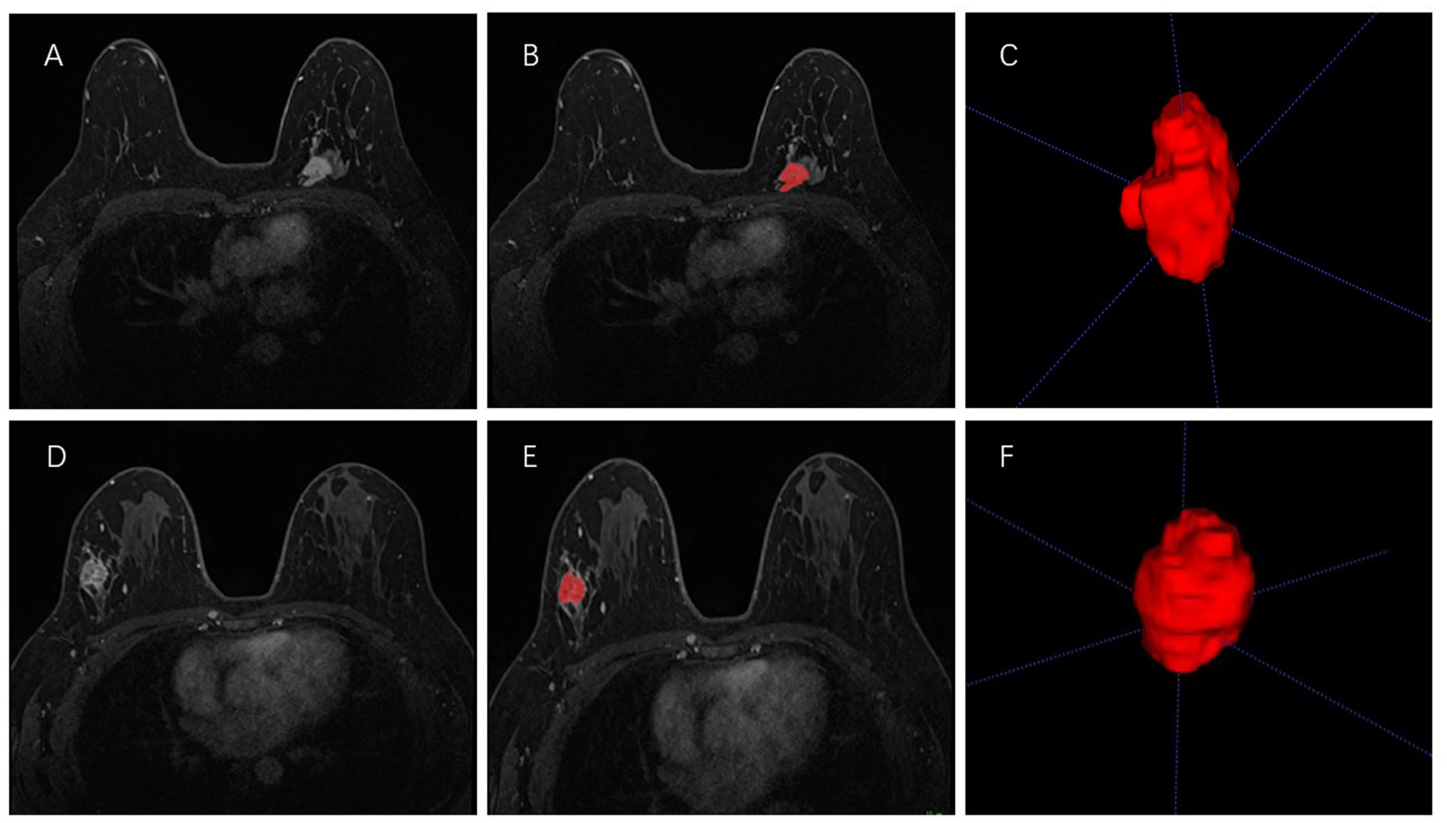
Manual 3-D segmentation of the tumor. (A–C): a 58-year-old female diagnosed with the luminal A subtype of breast cancer. (D–F): a 30-year-old female diagnosed with the triple-negative subtype of breast cancer. No special radiological features are seen by naked eye on MRI to distinguish between these two molecular subtypes.
